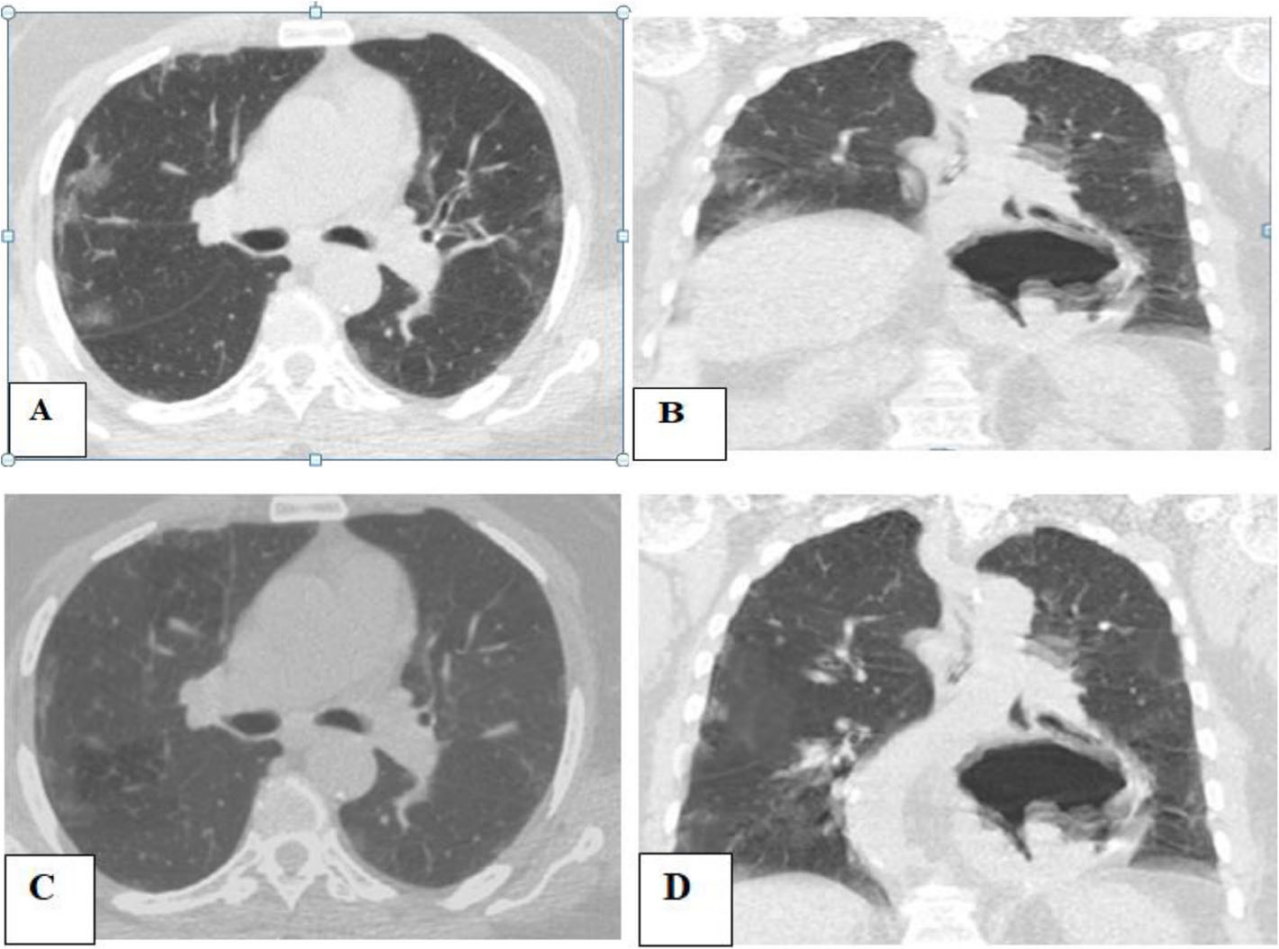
Fifty-one-year-old male patient, with a history of COVID-19 pneumonia, referred for initial CT evaluation with CT severity score 8. A, B Axial and coronal CT chest imaging respectively shows bilateral sub-pleural ground-glass patches and pulmonary consolidation with interlobar septal thickening. Incidental discovery of right-sided diaphragmatic paralysis. A notice of large axial hiatus hernia. C, D Images after 4 weeks reveals remarkable regression of bilateral patchy ground glassing and consolidation with resolution of the previous right phrenic paralysis
